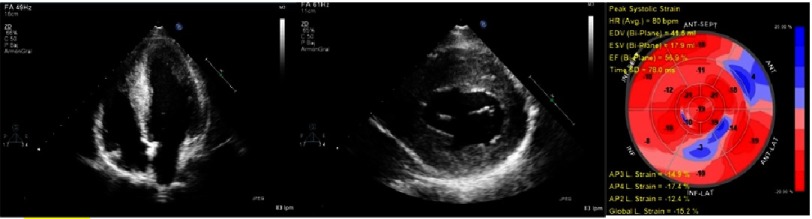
Echocardiography from a FA patient with HCM. The images show an apical 4 chamber with left ventricle HCM (mid-septal and apical regions) and left ventricle short axis image with concentric HCM. The last image shows regional dysfunction and decreased longitudinal strain analyzed with speckle-tracking myocardial strain.
